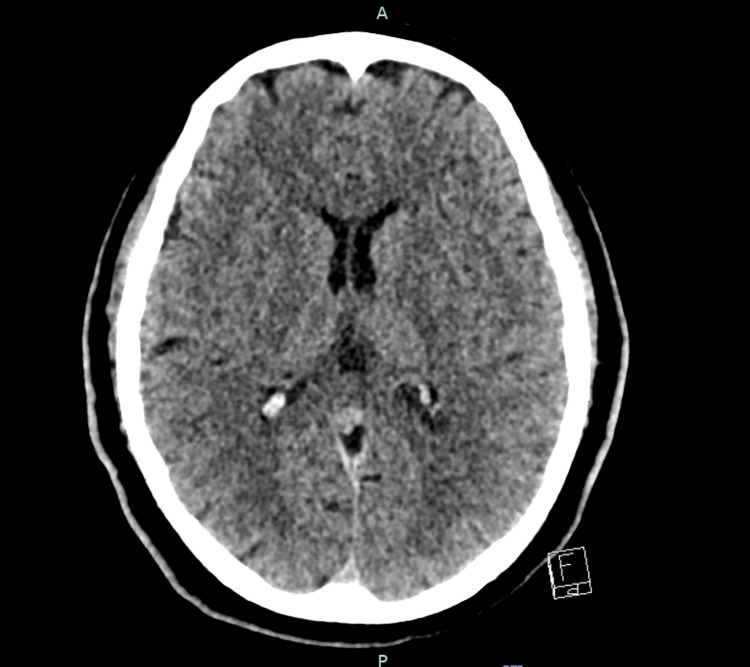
Non-contrast axial CT brain at the level of the basal ganglia demonstrating no acute intracranial abnormality
